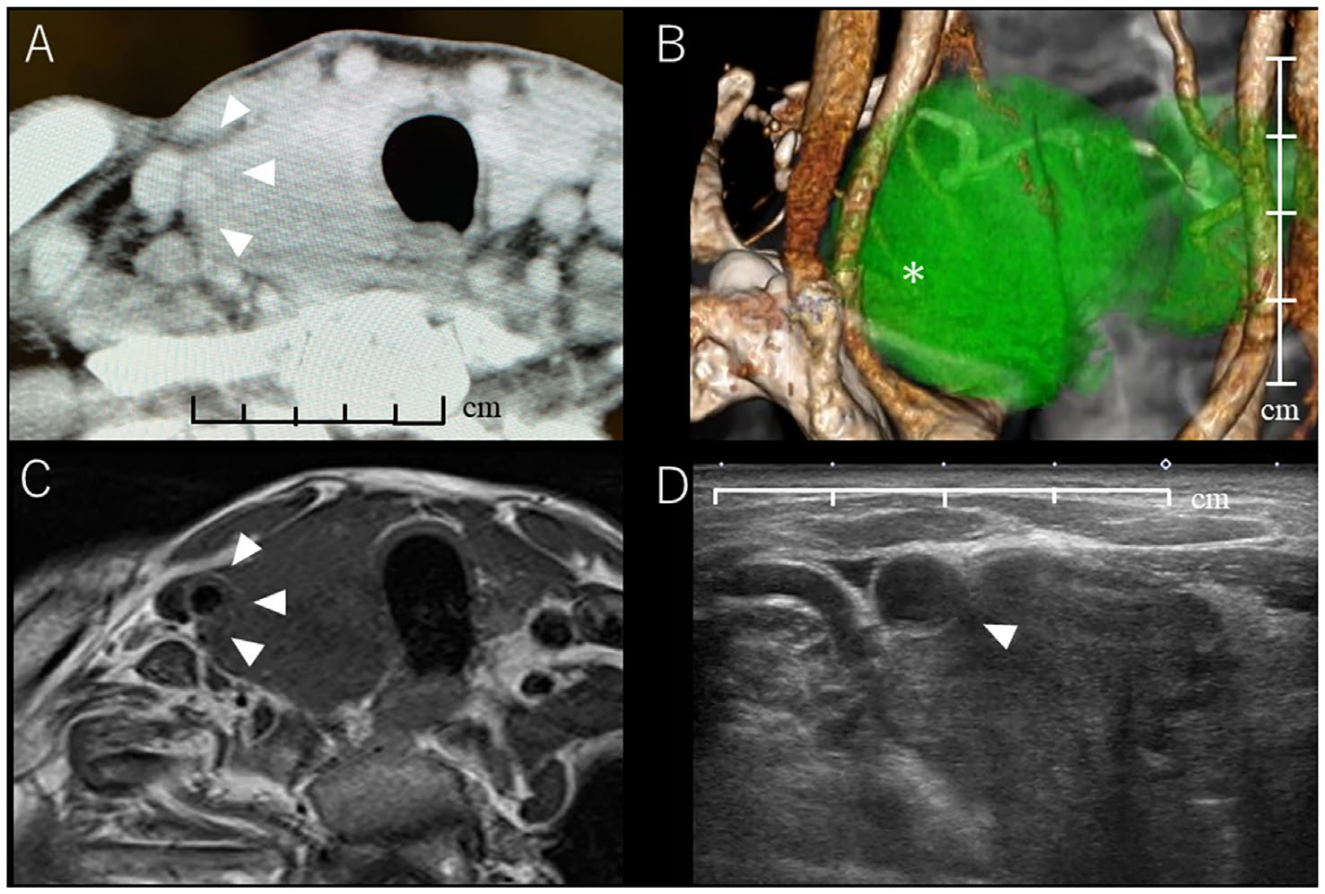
Preoperative images. (A) Preoperative CT axial image. The tumor was in contact with the common carotid artery for half of its circumference. The boundary was unclear in some areas (arrowhead). (B) 3D CT image. The common carotid artery was compressed by a tumor (asterisk), which appears to partially surround it. (C) T1‐weighted MRI image. Similarly, the area between the tumor and blood vessels is unclear (arrowhead). (D) Ultrasound image. The boundary between the outer membrane and middle membrane of the common carotid artery and the tumor is partially unclear (arrowhead). 3D, three dimensional; CT, computed tomography; MRI, magnetic resonance imaging.
